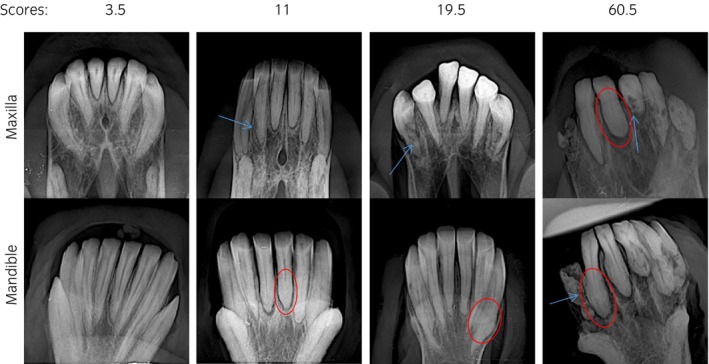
Representative radiographs showing the progression of EOTRH in four representative patients. Top row shows the maxillary teeth and the bottom row shows the mandibular teeth. Their score was devised from the adjusted radiographic scoring system of Albers et al.^17^ Blue arrows indicate tooth resorption and red circles indicate hypercementosis.
